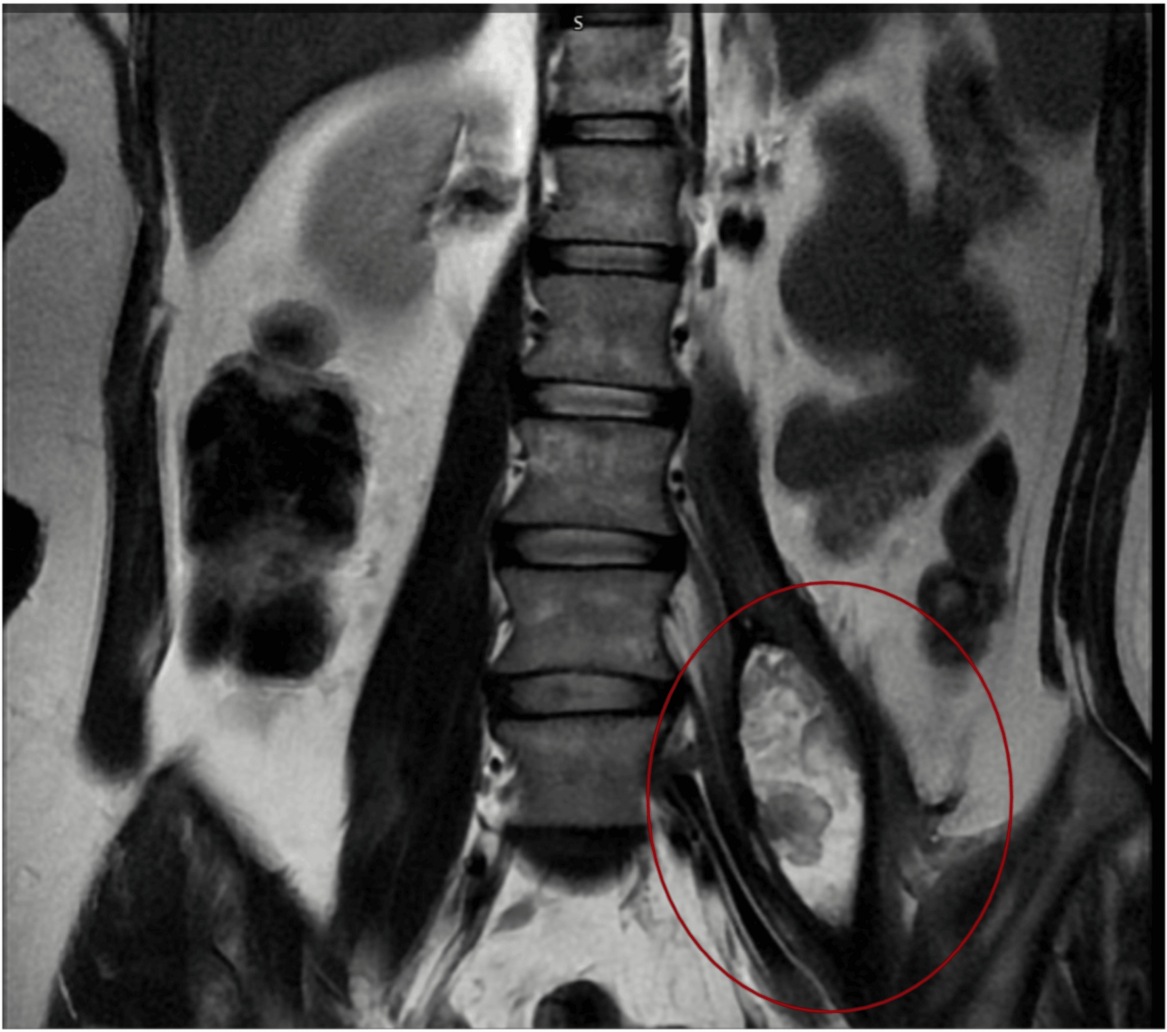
Iliopsoas hematoma diagnosed by a lumber contrast magnetic resonance imaging (MRI) in a T2 sequence during the six-month follow-up Coronal section shows a residual volume of 43 cc with heterogeneous content demonstrating calcification characteristics; no alteration is observed in adjacent abdominal or spinal structures. Cc: cubic centimeters
